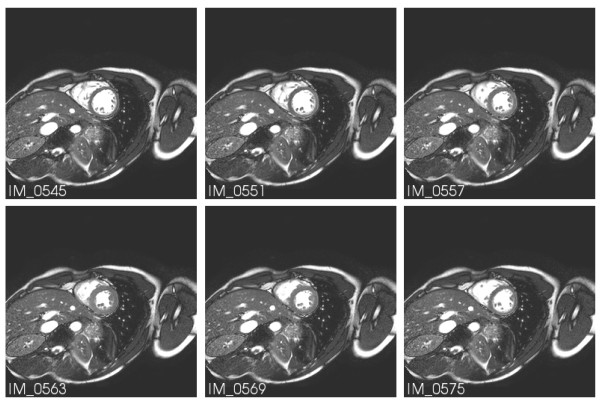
MR images at different heart cycle phases. MR images of Series 601 for the time instances marked in Figures 7 and 9 by red cursors.
